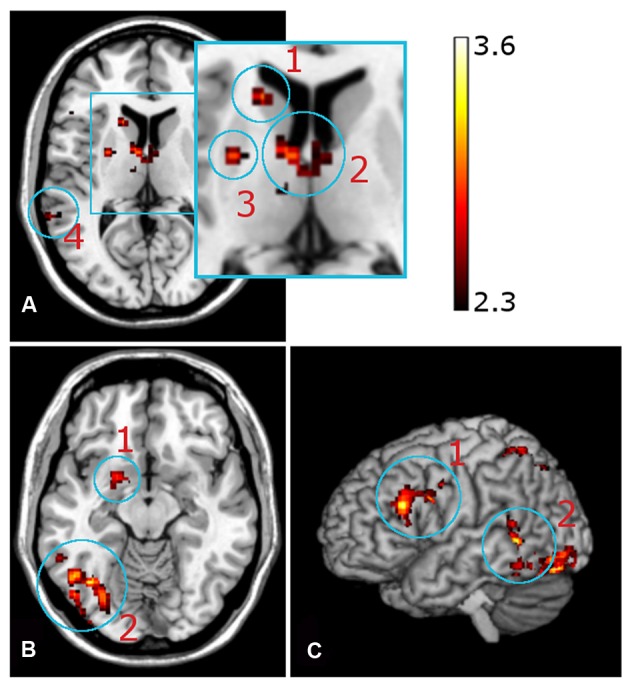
Functional magnetic resonance imaging contrast for successfully encoded (later recognized) > unsuccessfully encoded (later forgotten) CRA solutions. (A) Axial view, *z* = 10, (a1) left caudate nucleus, (a2) anterior thalamus, (a3) putamen, (a4) inferior temporal gyrus. (B) Axial view, *z* = -12, (1) amygdala, (b2) inferior temporal gyrus. (C) Sagittal view. (c1) inferior frontal gyrus, (c2) inferior/medial temporal gyrus.
